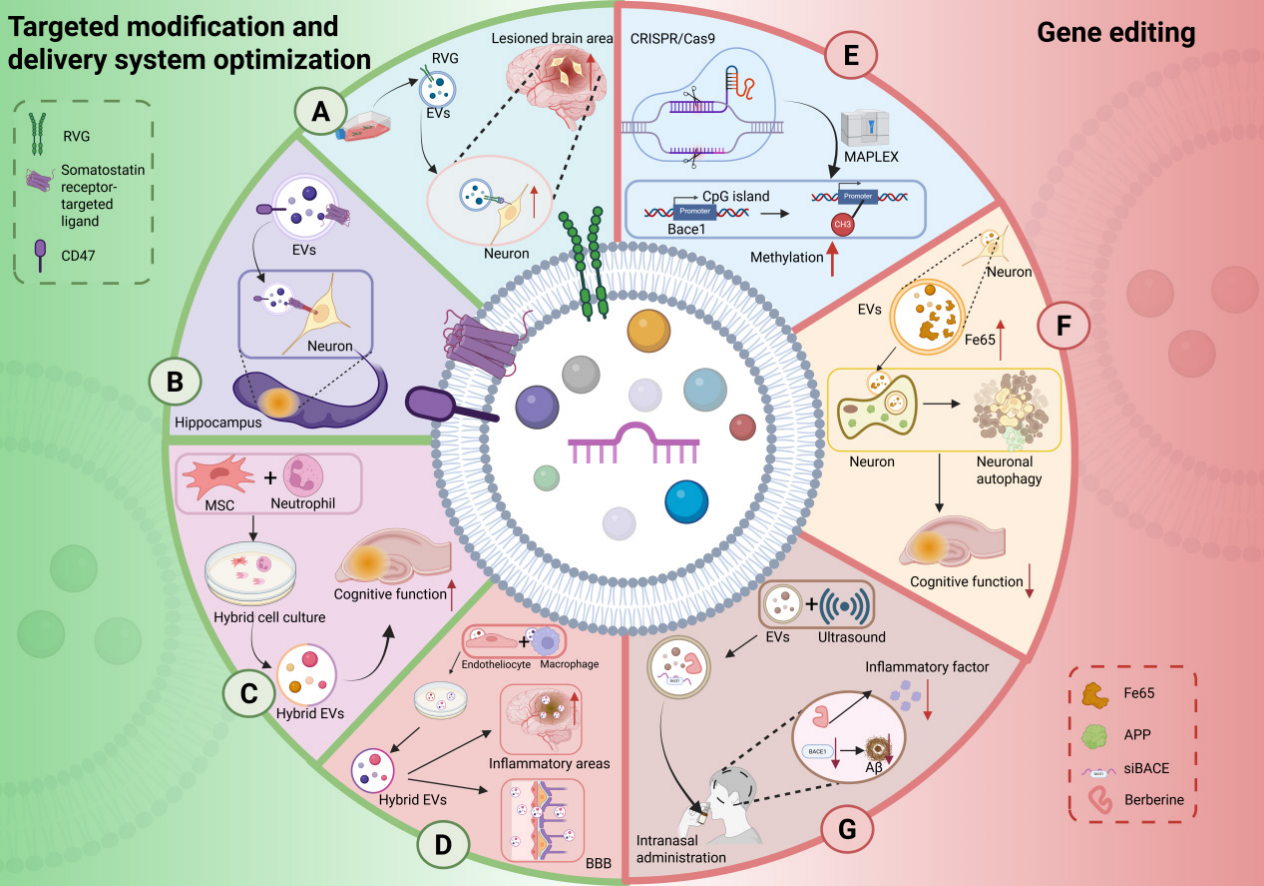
EV engineering strategies: targeted modification and gene editing. (A) Surface modification with RVG peptide enhances neuron-specific binding of EVs; (B) Expression of somatostatin receptor ligands enables hippocampus-specific EV delivery, while loading of CD47 ligands reduces their immune clearance and prolongs circulatory half-life; (C) EVs derived from mesenchymal stem cell-neutrophil hybrid cells target brain regions affected by AD; (D) Hybrid EVs generated by fusing membranes from brain microvascular endothelial cells and macrophage-derived EVs exhibit dual capabilities, which can efficiently cross the blood-brain barrier and accumulate in the inflamed areas of the brain, promoting the clearance of Aβ; (E) The CRISPR/Cas9 system is utilized to epigenetically target and methylate the BACE1 promoter, thereby reducing its expression; (F) Engineered hippocampal neuron-derived EVs overexpressing the Fe65 protein induce autophagy in APP-expressing neurons; (G) Ultrasound-mediated preparation generates EVs co-loaded with BACE1 siRNA and the anti-inflammatory drug berberine, enabling synergistic therapy upon intranasal administration. RVG: Rabies virus glycoprotein; EV: extracellular vesicle; MSC: mesenchymal stem cell; BBB: blood-brain barrier; MAPLEX: mMaple3-mediated protein loading into and release from exosomes; APP: amyloid precursor protein; Aβ: amyloid-beta; BACE1: beta-site amyloid precursor protein cleaving enzyme 1; CRISPR: clustered regularly interspaced short palindromic repeats; Cas9: CRISPR-associated protein 9; siRNA: small interfering RNA. Created in BioRender. yinghan, h. (2025) https://BioRender.com/br23jn3
